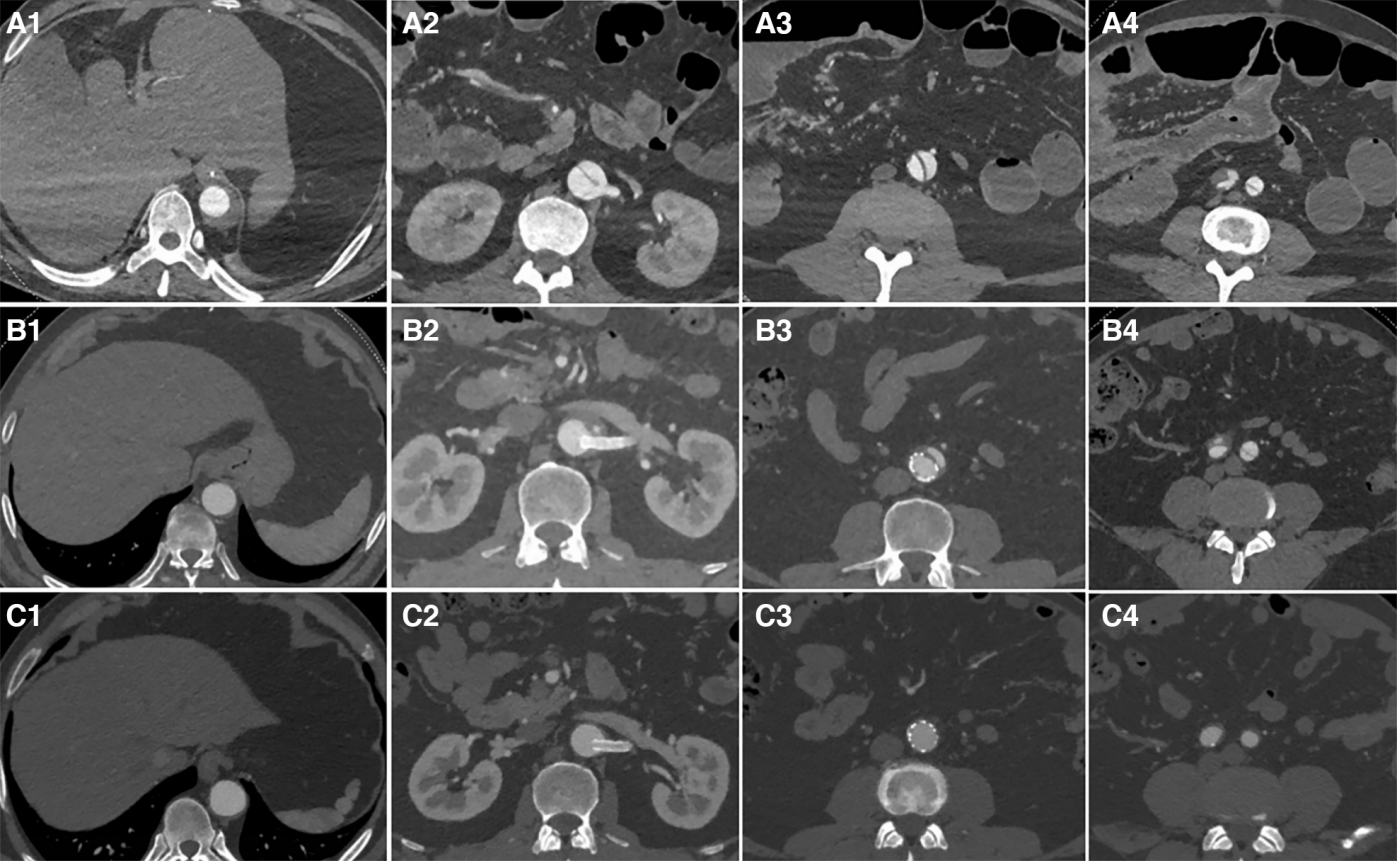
The first row displays preoperative CT images, the second row presents CT images taken three months after the second-stage surgery, and the third row features follow-up CT images taken one year after the second-stage surgery. From left to right, the images respectively show sections of aortic dissection at the distal end plane of the primary stent, the renal artery level, 5 cm below the renal artery level, and 1 cm below the bifurcation of the iliac artery. After initial surgery, zones (A–D) exhibited a residual FL that had not fully thrombosed (A1-A4). Three months after the second-stage surgery, the aortic CTA revealed TL re-expansion without any obvious entry tears; follow-up observation was subsequently continued (B1-B4). One year after second-stage surgery, aortic CTA revealed that the FL had completely thrombosed and disappeared (C1-C4).
